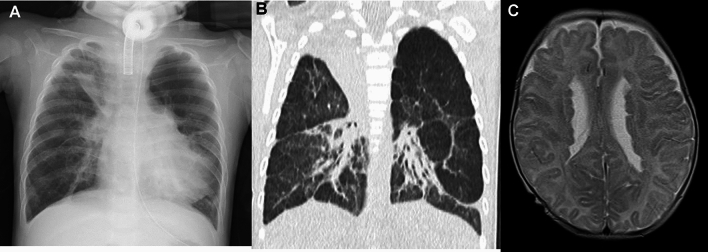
FLNA*-associated ILD. A 5-month-old girl with biallelic *FLNA* mutations. A,B CXR and CT showed bilateral emphysematous changes associated with subsegmental atelectases predominating in the upper lungs. C T2-weighted brain MRI displayed periventricular nodular heterotopia
